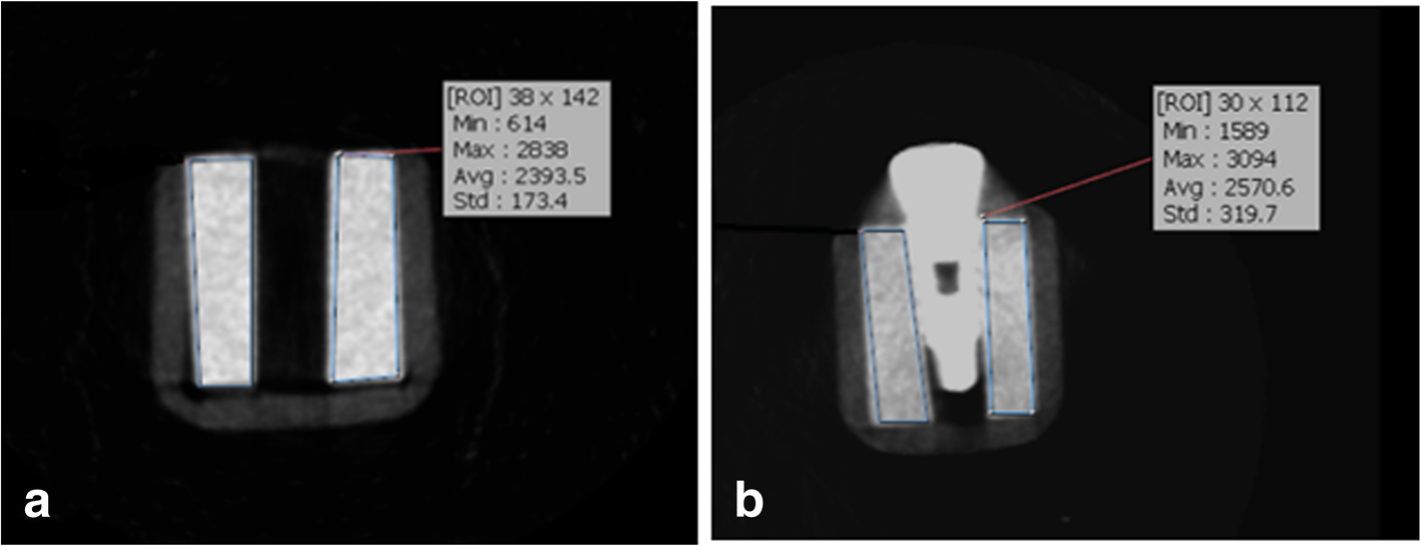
CBCT scans of the samples for type 1 bony density before (a) and after (b) insertion of implants in this exposure parameters: FOV: 4 × 6 cm^2^, mA: 4, T:6.1 s, kVp:90
